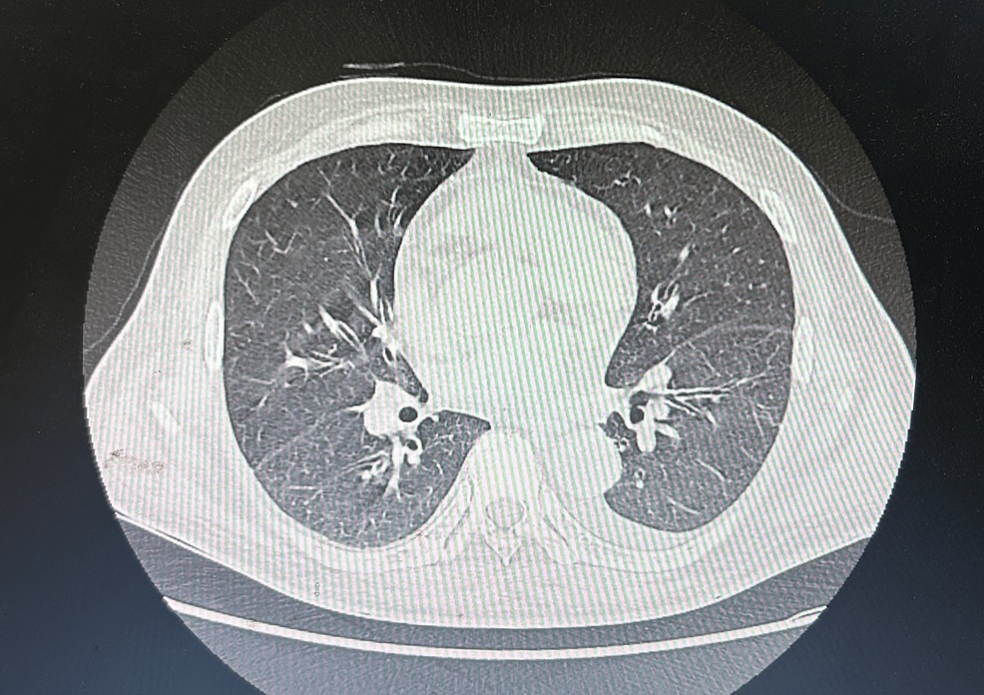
Chest CT Chest CT showed no obvious abnormalities in the lungs but revealed a small amount of pleural effusion.
